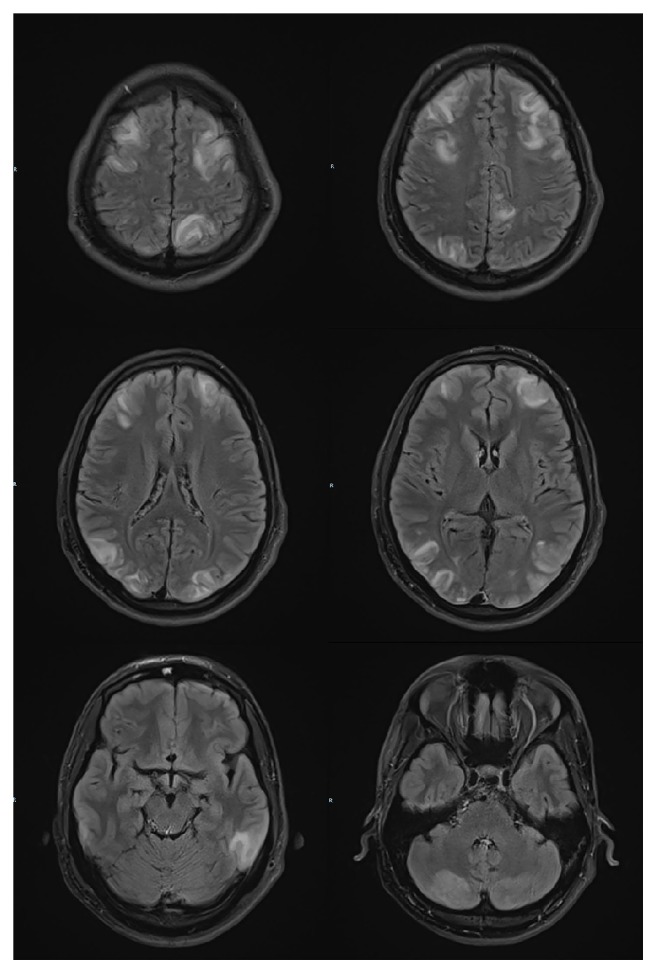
T2 FLAIR sequence. MRI brain (done on Day 4 of heat stroke) showed widespread T2 FLAIR hyperintensities over both cerebral and cerebellar hemispheres, predominantly the cortex and the subcortical white matter.
